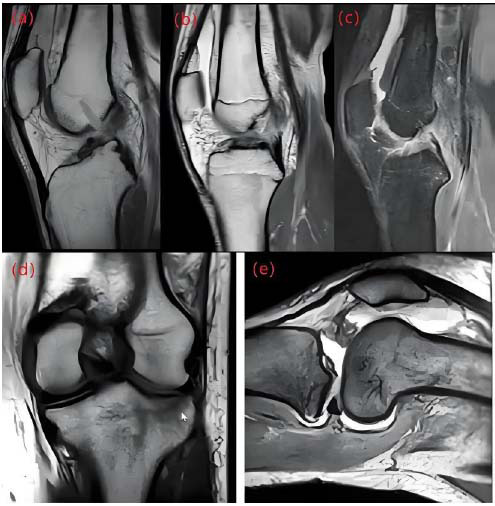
MRI images of knee joint.
